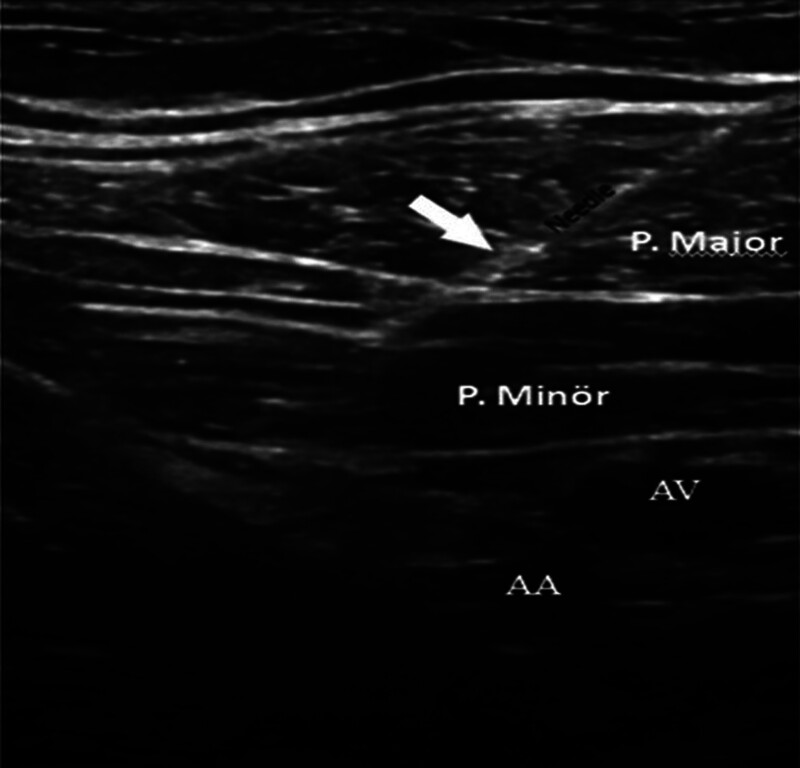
An ultrasound image of pectoralis minor muscle block. Arrow-head indicates the needle. AA = axillary artery, AV = axillary vein, P. Major = pectoralis major muscle, P. Minör = pectoralis minor muscle.
